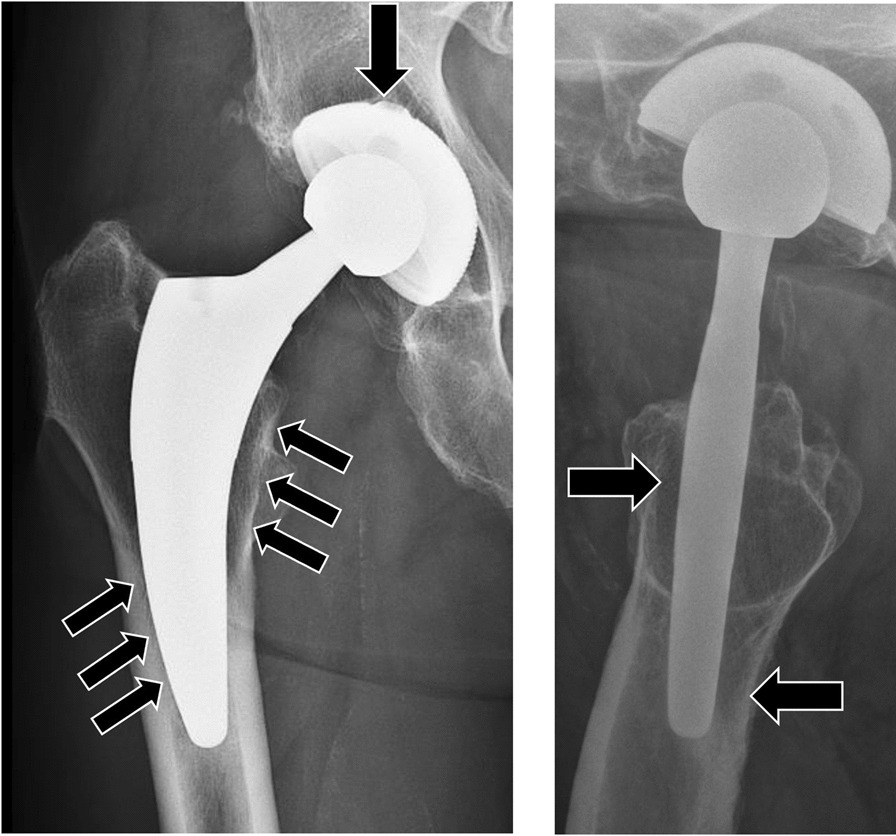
Radiographs anteroposterior (ap) and axial of the Fitmore hip stem position in the femoral shaft. Load transfer contact points at the implant-bone interface indicated via arrows at the femoral head element; calcar; and anterior/posterior subtrochanteric shaft
